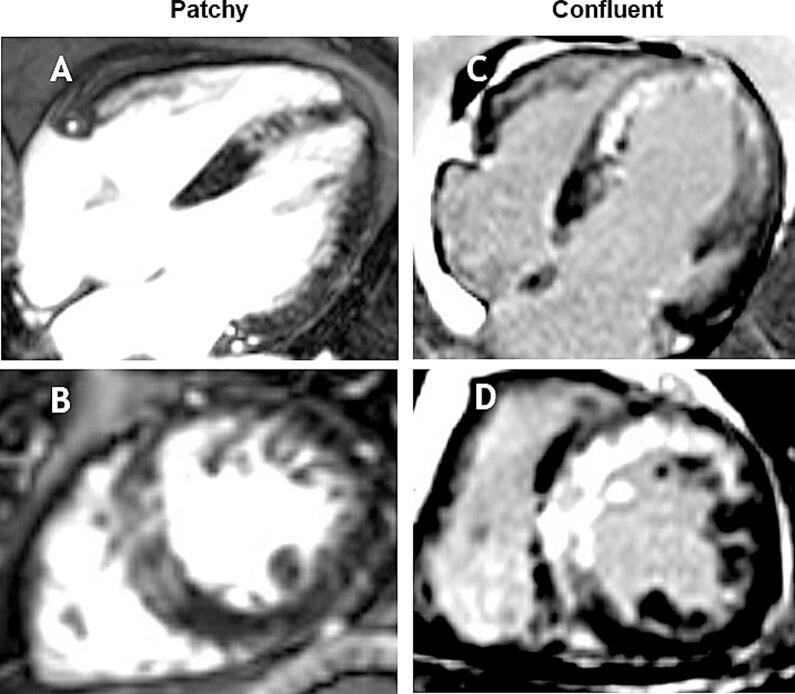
Example assessment LGE pattern*.* A, B: CMR-LGE acquisition of the AP4CH view and SAX view demonstrating patchy infarction. C,D: CMR-LGE acquisition of the AP4CH view and SAX view demonstrating confluent infarction. Abbreviations: AP4CH = apical 4-chamber view; CMR = cardiovascular magnetic resonance; LGE = late gadolinium enhancement; SAX = short axis.
